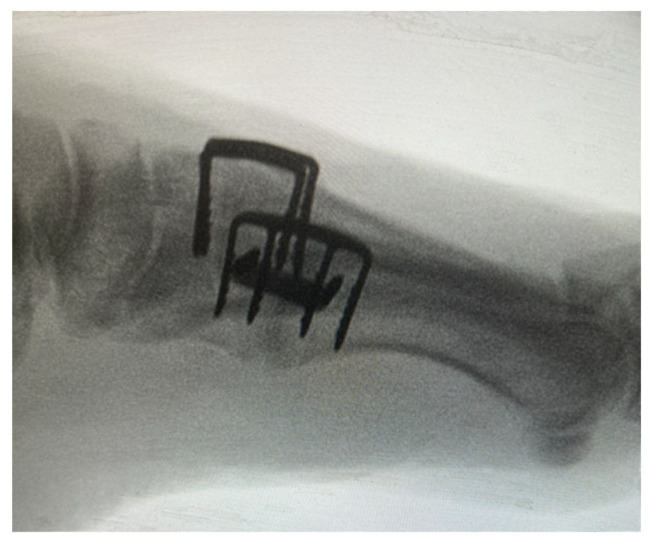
Lateral intra-operative radiograph depicting a 1st/2nd/3rd tarsometatarsal joint fusion with a 90-90-degree configuration technique for the 1st TMTJ.
